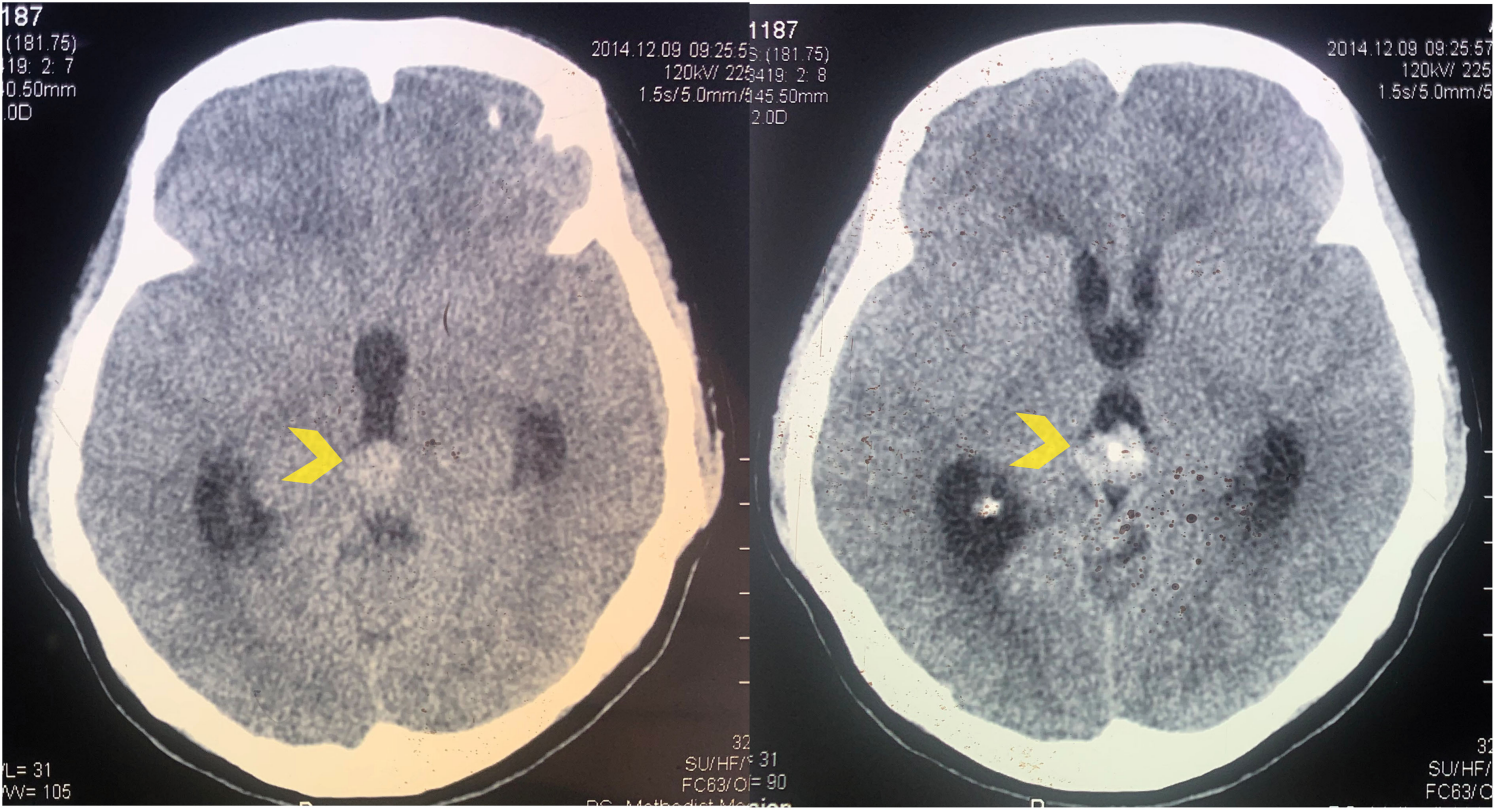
Axial CT scan without contrast showing the pineal region tumor at the time of the initial diagnosis in 2014 (yellow arrowhead).
